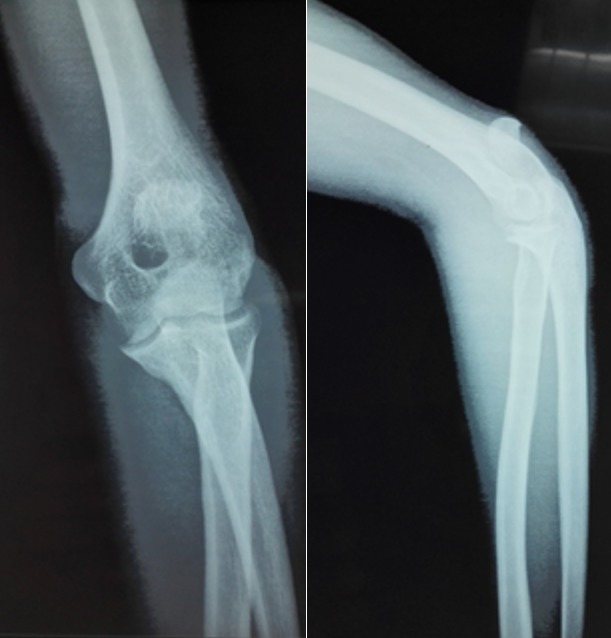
radiographie du coude de face et de profil
